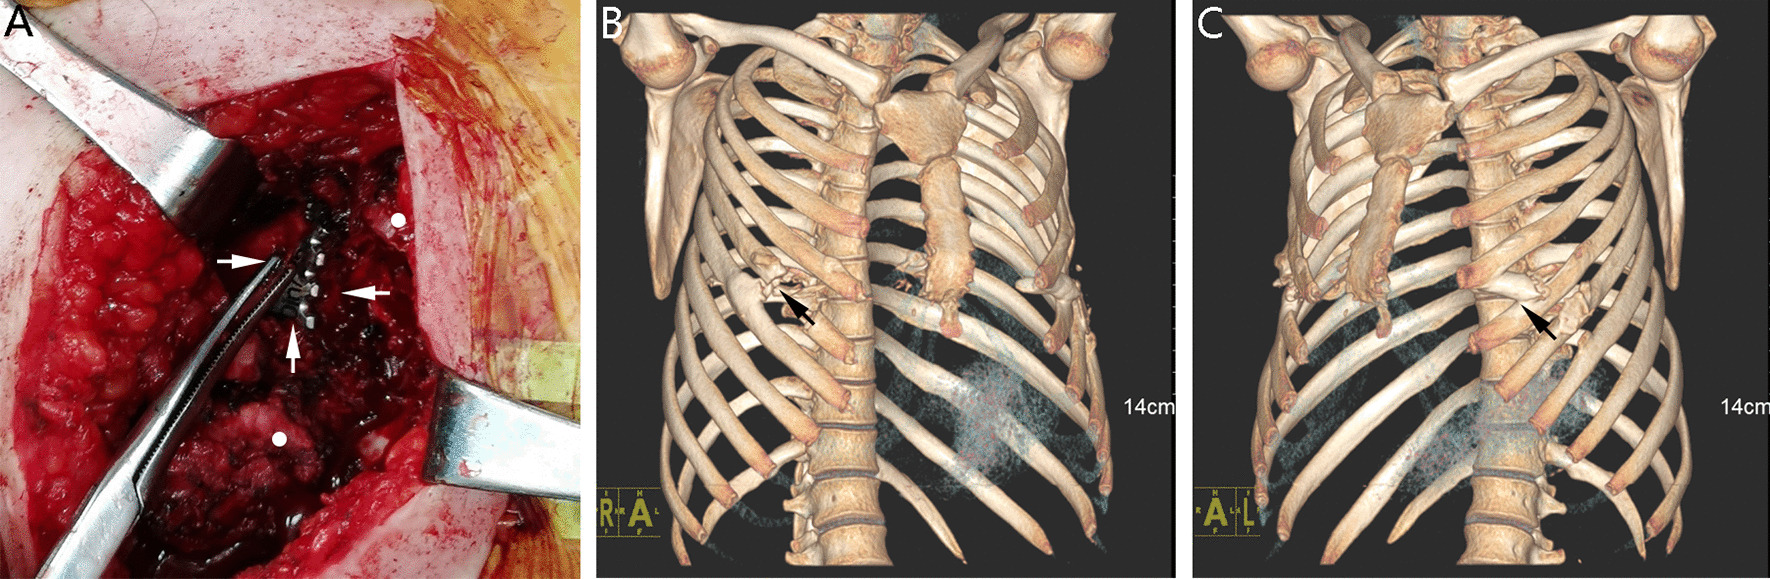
A The right end of the lower bar (upward arrow) had shifted into the thoracic cavity (rightward arrow). Large amounts of hyperplastic bone tissue (leftward arrow) was on the inner side of the ribs. Two broken ends (round spot) left after partial excision of the fifth rib. B and C Three-dimensional reconstruction of rib CT demonstrated the significant rib periosteal hyperplasia from different angles, forming the sheath-like structure around the bars(tilted arrow). The longitudinal axis of the sternum was twisted to the right
